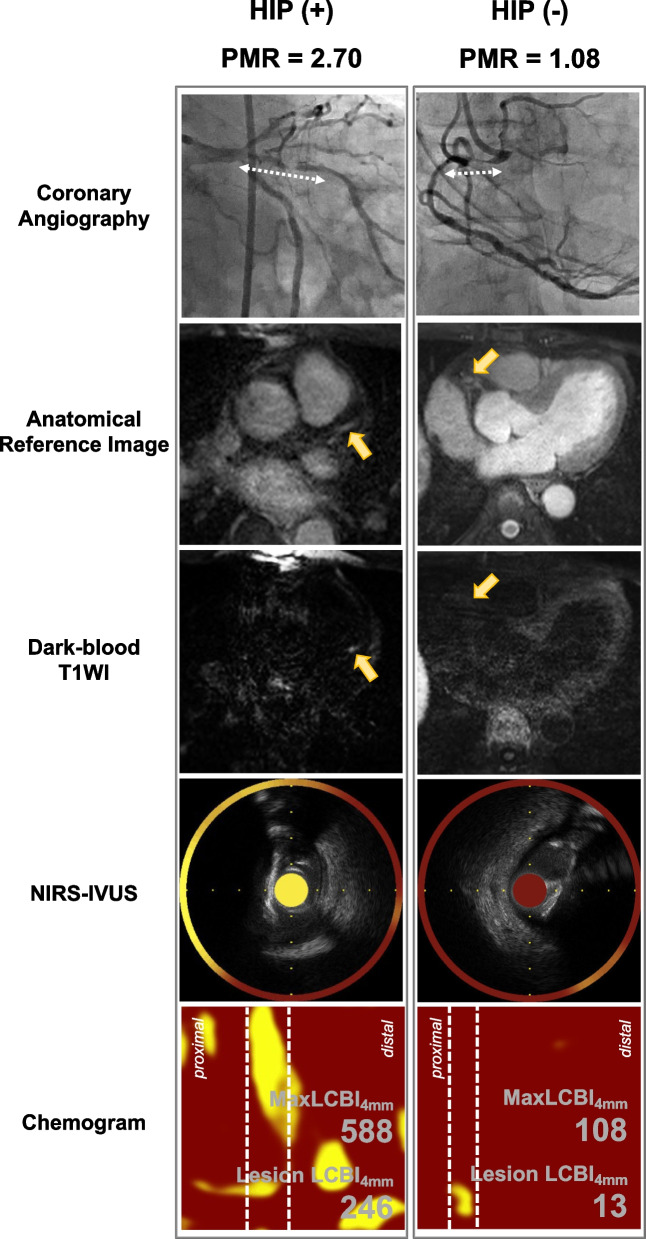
Representative images of coronary culprit lesions with calcified plaque. The left panel shows the images of a patient with HIP and calcified plaque, and the right panel shows the images of a patient with non-HIP and calcified plaque. A culprit lesion site is detected on coronary angiography (white dotted arrow). After identifying the same site using an anatomical reference image, the presence of HIP is confirmed using dark-blood T1-weighted imaging (yellow arrow). In addition, NIRS-IVUS shows a calcified plaque and maximum LCBI at the 4-mm segment using a chemogram. T1w, T1 weighted image
